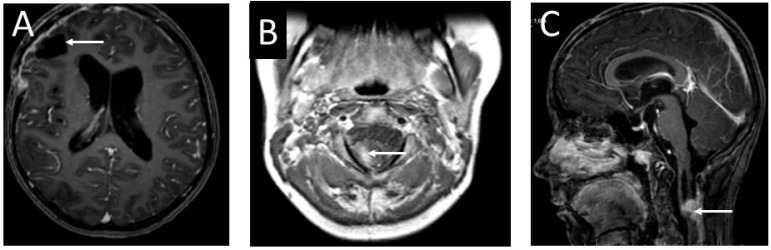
Contrast-enhanced T1 MRI images of the head and cervical spine of the 13-year-old girl before the valve implantation: (A) an axial section of the head. The photo does not show progression of the tumor (arrow). (B) MRI of the cervical spine—an axial section. Visible remnants of the tumor diagnosed as melanocytoma (arrow). (C) An MRI—sagittal section of the cervical spine. The residual tumor mass shows no enlargement compared to previous diagnostic images (arrow).
